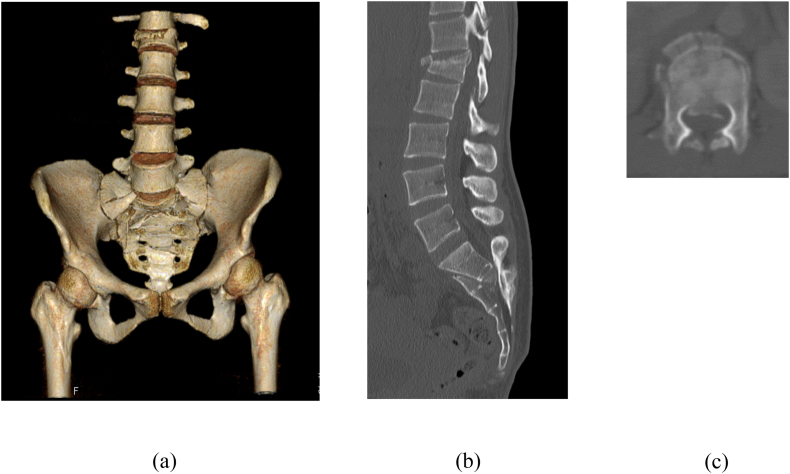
(a) Three-dimensional computed tomography (CT) showed an H-shaped Roy-Camille type II sacral fracture and a L1 burst fracture on admission. (b) Sagittal CT demonstrated the displaced fragment obstructing the sacral canal at the S2 level. (c) Axial CT showed 30% spinal canal compromise at the L1 level.
